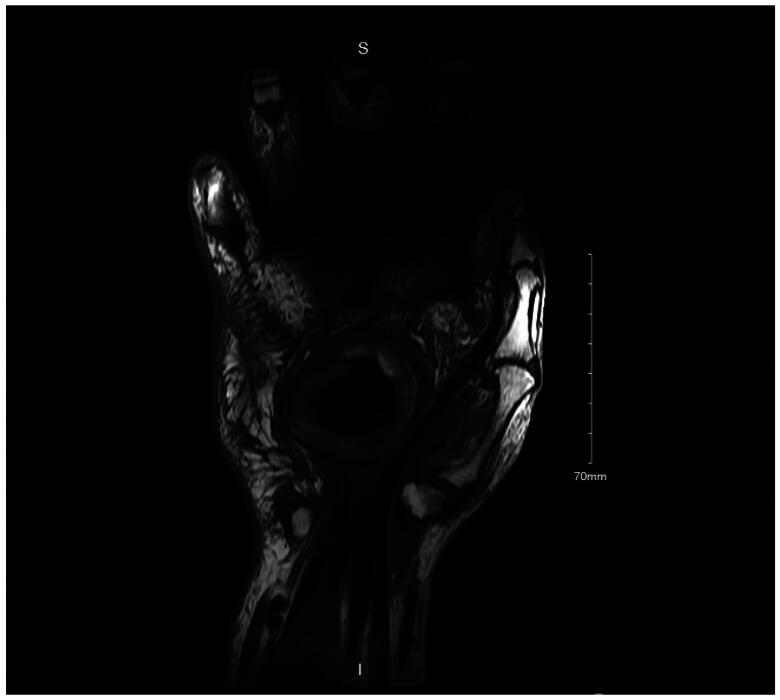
Axial MRI of the left hand.
